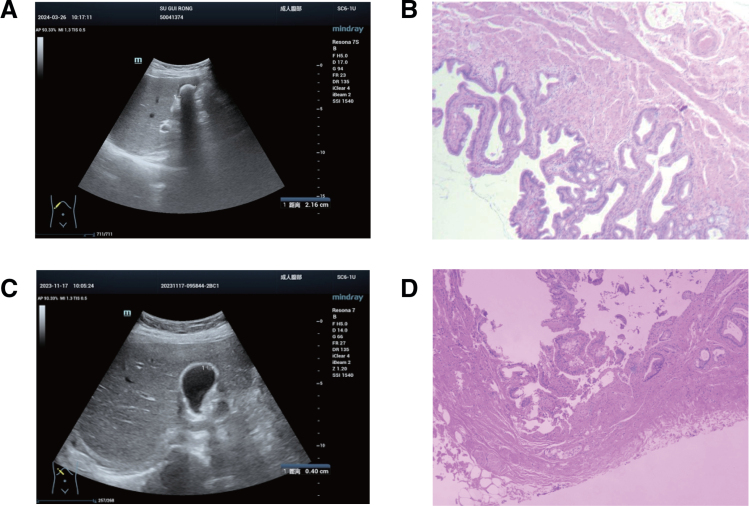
Ultrasound and pathological images of patients. (A) Ultrasound image of a patient with cholelithiasis; (B) Pathology image of a patient with cholelithiasis. (C) Ultrasound image of a patient with cholecystic polyps. (D) Pathology image of a patient with cholecystic polyps.
